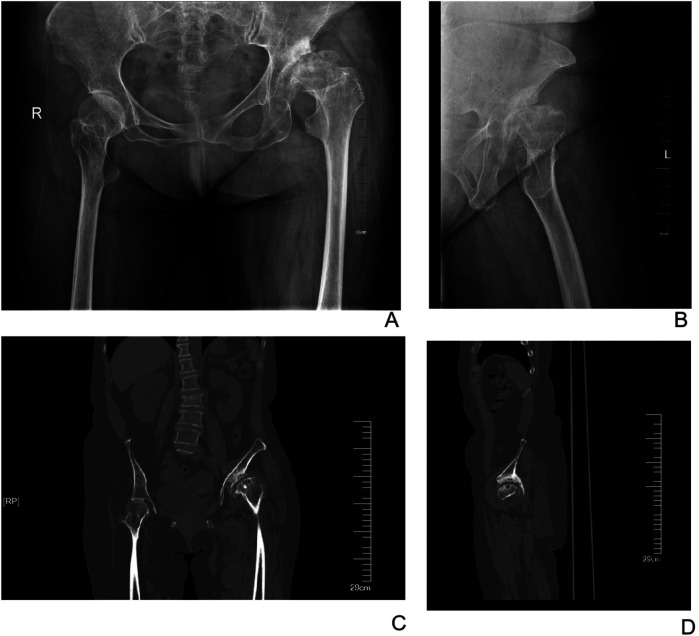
Preoperative X-ray and CT images of a 60-year-old female patient. (A) Anteroposterior radiograph of the hips. (B) Lateral radiograph of the left hip. (C) Anteroposterior CT image of the hips. (D) Lateral CT image of the left hip.
